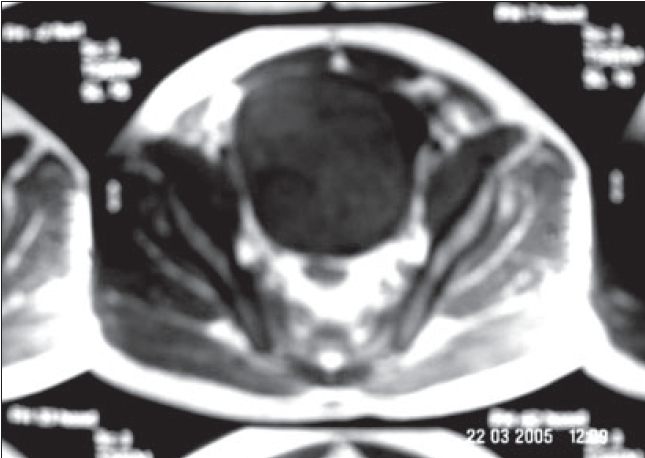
MRI- Well circumscribed intravesical mass
